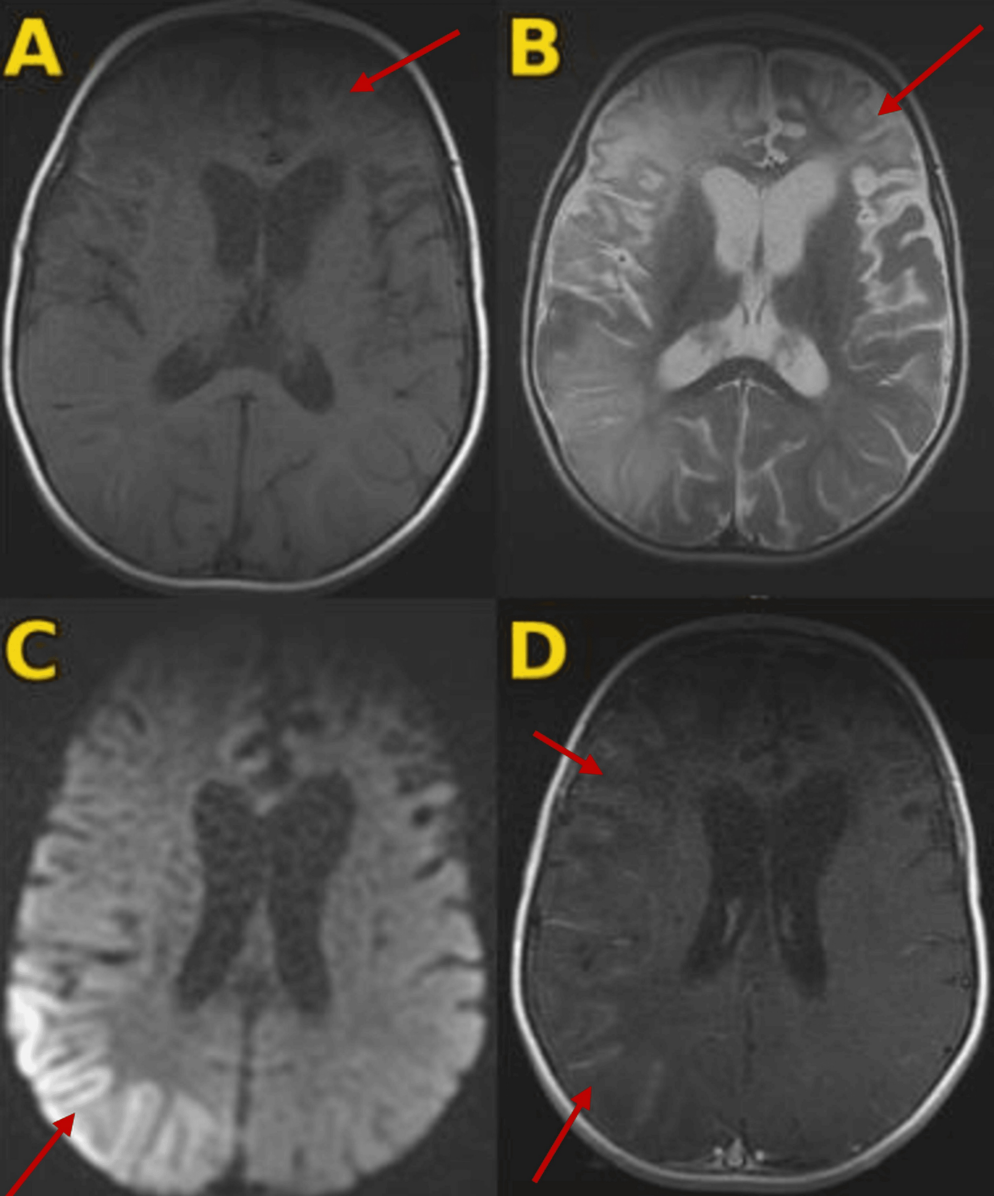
Brain MRI performed on day 3 of seizure onset A: Axial T1-weighted sequence showing a left frontal cortico-subcortical signal abnormality that is hypointense on T1, associated with enlargement of the ipsilateral frontal horn, consistent with chronic/post-injury sequelae (red arrow). B: Axial T2-weighted sequence showing the same left frontal cortico-subcortical lesion, hyperintense on T2, with ex vacuo dilatation of the ipsilateral frontal horn (red arrow). C: Diffusion-weighted imaging (DWI) demonstrating marked diffusion hyperintensity in the right parietal cortico-subcortical region involving the cortex and adjacent subcortical white matter; the pattern is non-territorial and does not conform to a specific arterial vascular territory (red arrow). D: Axial post-contrast T1-weighted image showing marked leptomeningeal enhancement in the right fronto-temporo-parietal region (red arrows).
